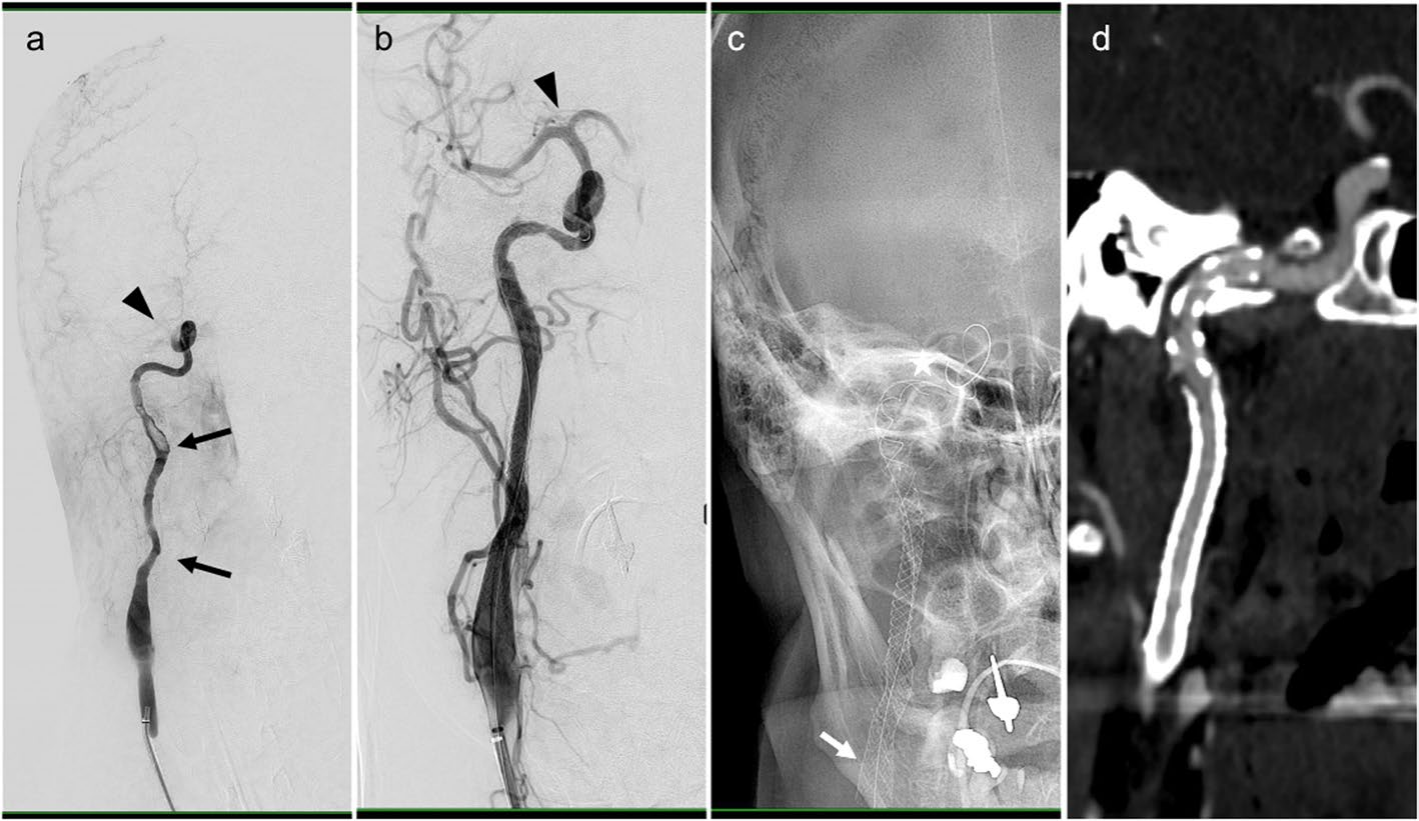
DSA images of a male patient with cervical ICA dissection (arrows in a) and carotid-T occlusion (arrowhead in a). The MCA was recanalized (arrowhead in b) and the ICA reconstructed using three overlapping stents (c: arrow shows proximal end, asterisk distal end). The patient was treated with i.v. Lysis and therefore received Aspirin only at the beginning of the intervention. Due to extensive clot formation, i.v. eptifibatide was started prior to stent placement. Control CTA 12 h post intervention showed patency of the stents (d). DSA: Digital subtraction angiography, ICA: Internal carotid artery, MCA: Middle cerebral artery, CTA: Computerized Tomography Angiography
